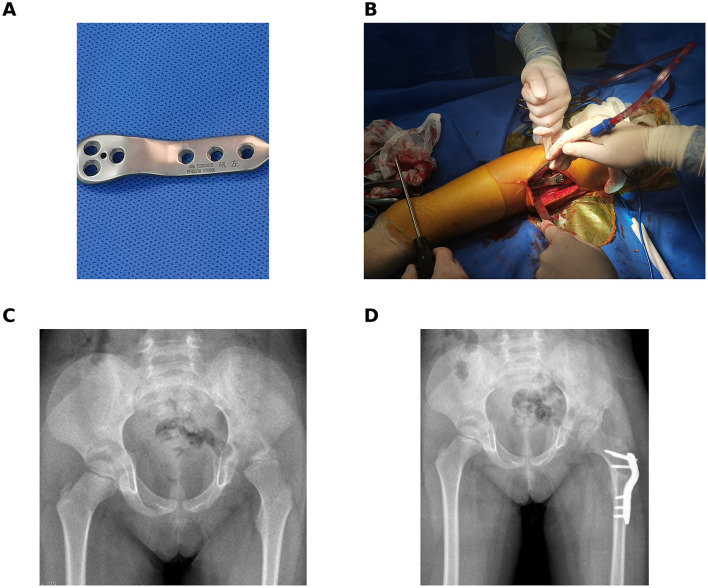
(A) Gross appearance of the customized plate. (B) Intraoperative use of the customized plate. (C) Preoperative X-ray of the patient. (D) Postoperative X-ray of the patient.
